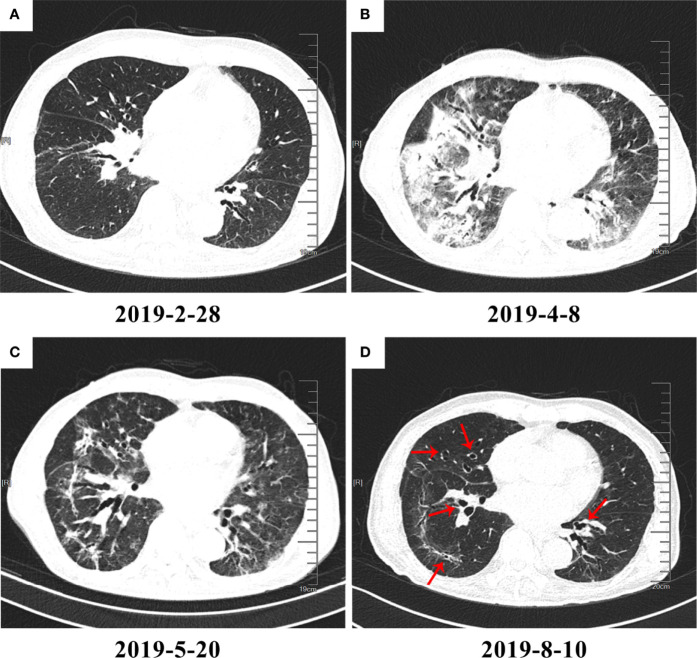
High-resolution computed tomography (HRCT) imaging of checkpoint inhibitor–related pneumonitis (CIP) in different phases. On March 1, 2019, the patient began immunotherapy and developed CIP on April 8, 2019. (A) Baseline HRCT image. (B) CIP in the acute phase. Diffuse patchy shadows and ground-glass opacities (GGOs) with consolidation can be seen in the image. (C) CIP in the subacute phase. The patchy shadows, GGOs, and consolidation appear to have been greatly absorbed. However, traction bronchiectasis can be seen developing to a severe degree. (D) CIP in the chronic phase. Most inflammation-related changes have been absorbed, and the lesions are mainly leftover traction bronchiectasis (red arrows).
